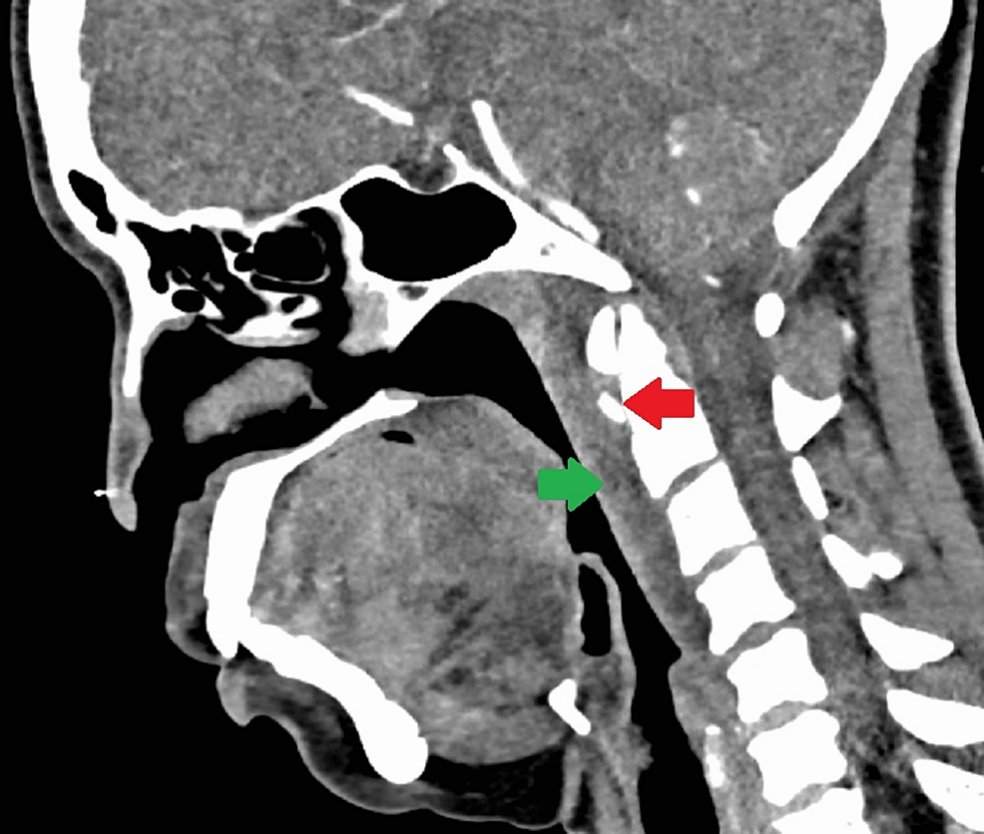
Contrast-enhanced computed tomography (CT) of the neck (sagittal view) Amorphous calcification is seen anterior to the dens (red arrow) associated with prevertebral effusion (green arrow).
